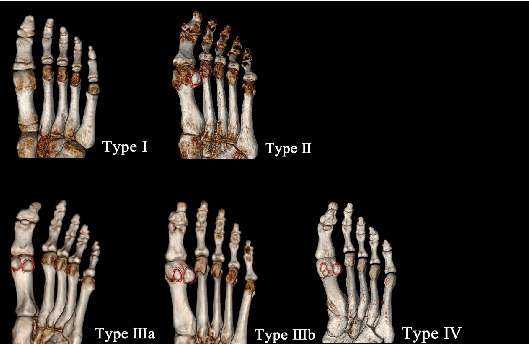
The types of HSBs on inferior by 3D CT image. Type I: no HSB; type II: with one HSB; type IIIa: with two HSBs when THB is bigger; type IIIb: with two HSBs when FHB is bigger; and type IV: with three HSBs. All HSBs are circled in red.
